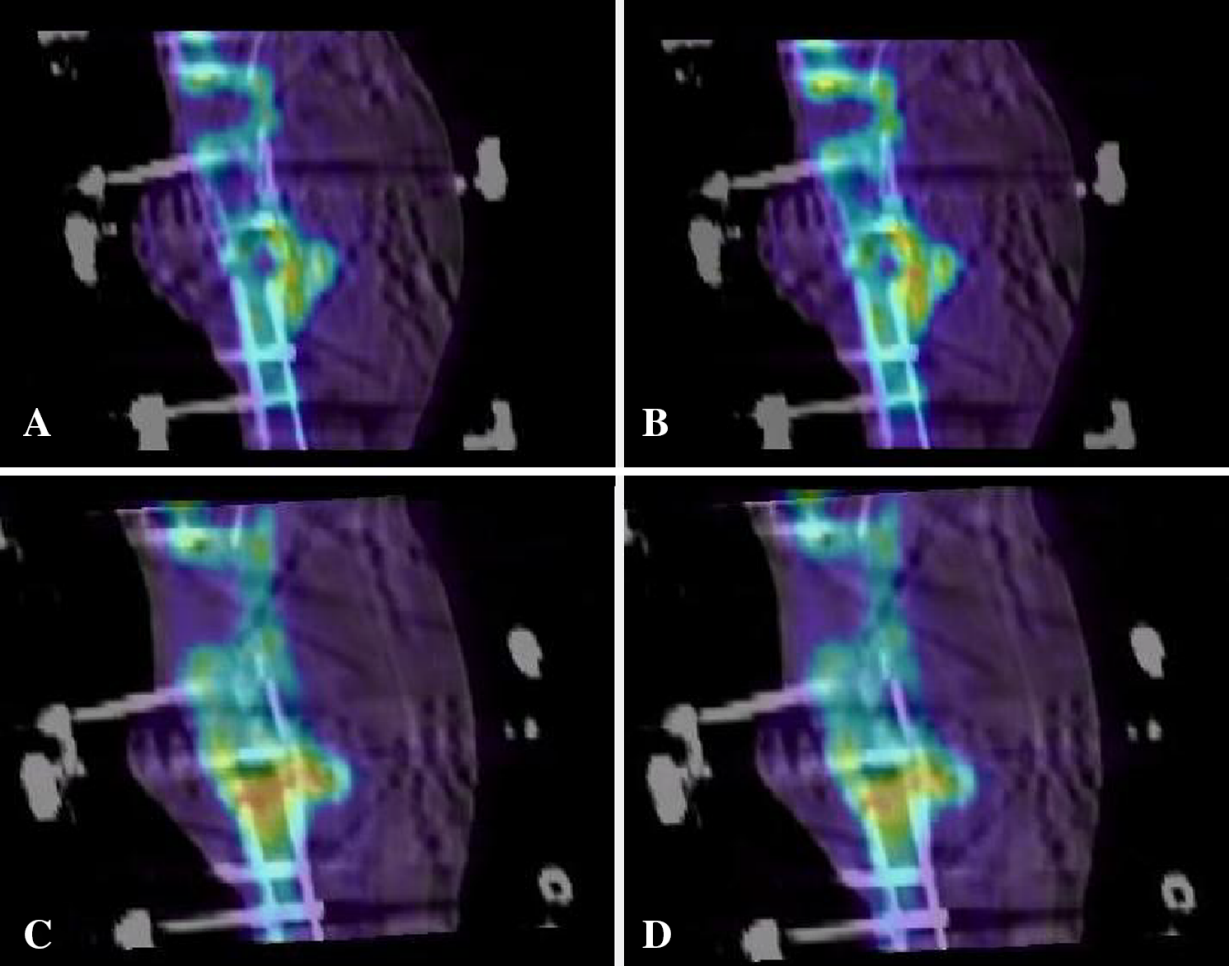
^18^F^−^ uptake is pictured for Patient 15. At the first 6-week scan before ultrasound stimulation, the uptake at (A) 20 minutes after injection and (B) 30 minutes after injection, appears uneven especially across the distal pseudarthrosis. At the 6-week scan after ultrasound stimulation, (C) 20 minutes after injection and (D) 30 minutes after injection, bone formation across the distal pseudarthrosis and the proximal osteotomy appears to be more uniform.
